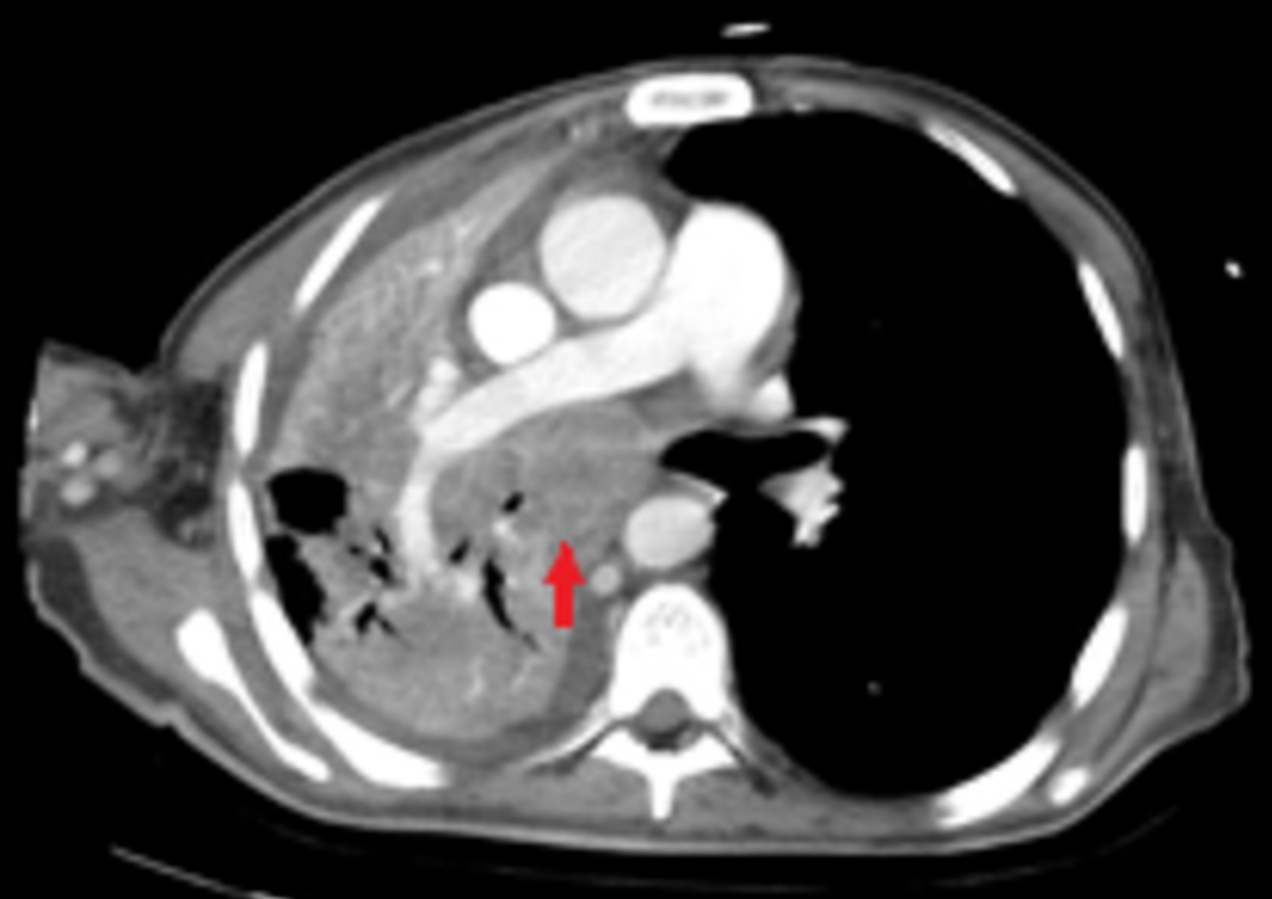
Computed tomography scan of the chest of the same area, at current presentation, showing a lung mass encasing the right upper lobe bronchus
